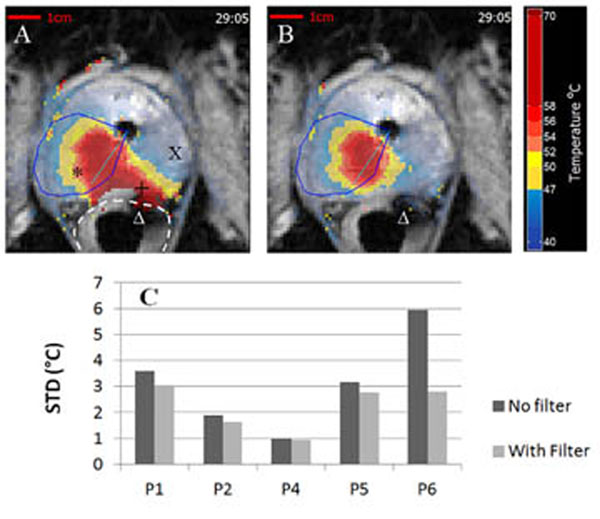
MR thermal maps without (A) and with filter (B), during transurethral ultrasound therapy with moving air bubble in the rectum (Δ) inducing a temperature artifact (+) that interfered with the real heating (*). Average STD over the Rectum zone by patient.
